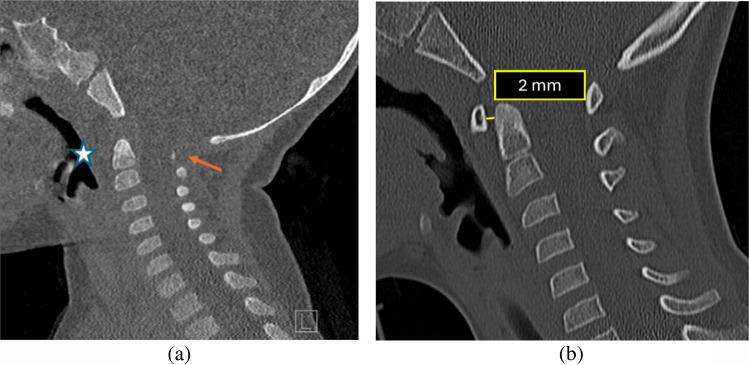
Sagittal CT in a 10-month-old with suspected non-accidental trauma. (a) The anterior arch of the atlas is not yet ossified (*star*). Minimally ossified posterior arch of C1 is seen (*orange arrows*). In these cases,ADI cannot be measured accurately, and clinical suspicion along with secondary signs of injury on soft-tissue windows may be useful. (b) Sagittal CT in a 7-year-old post-trauma shows a normal ADI (normal <2.6 mm)
